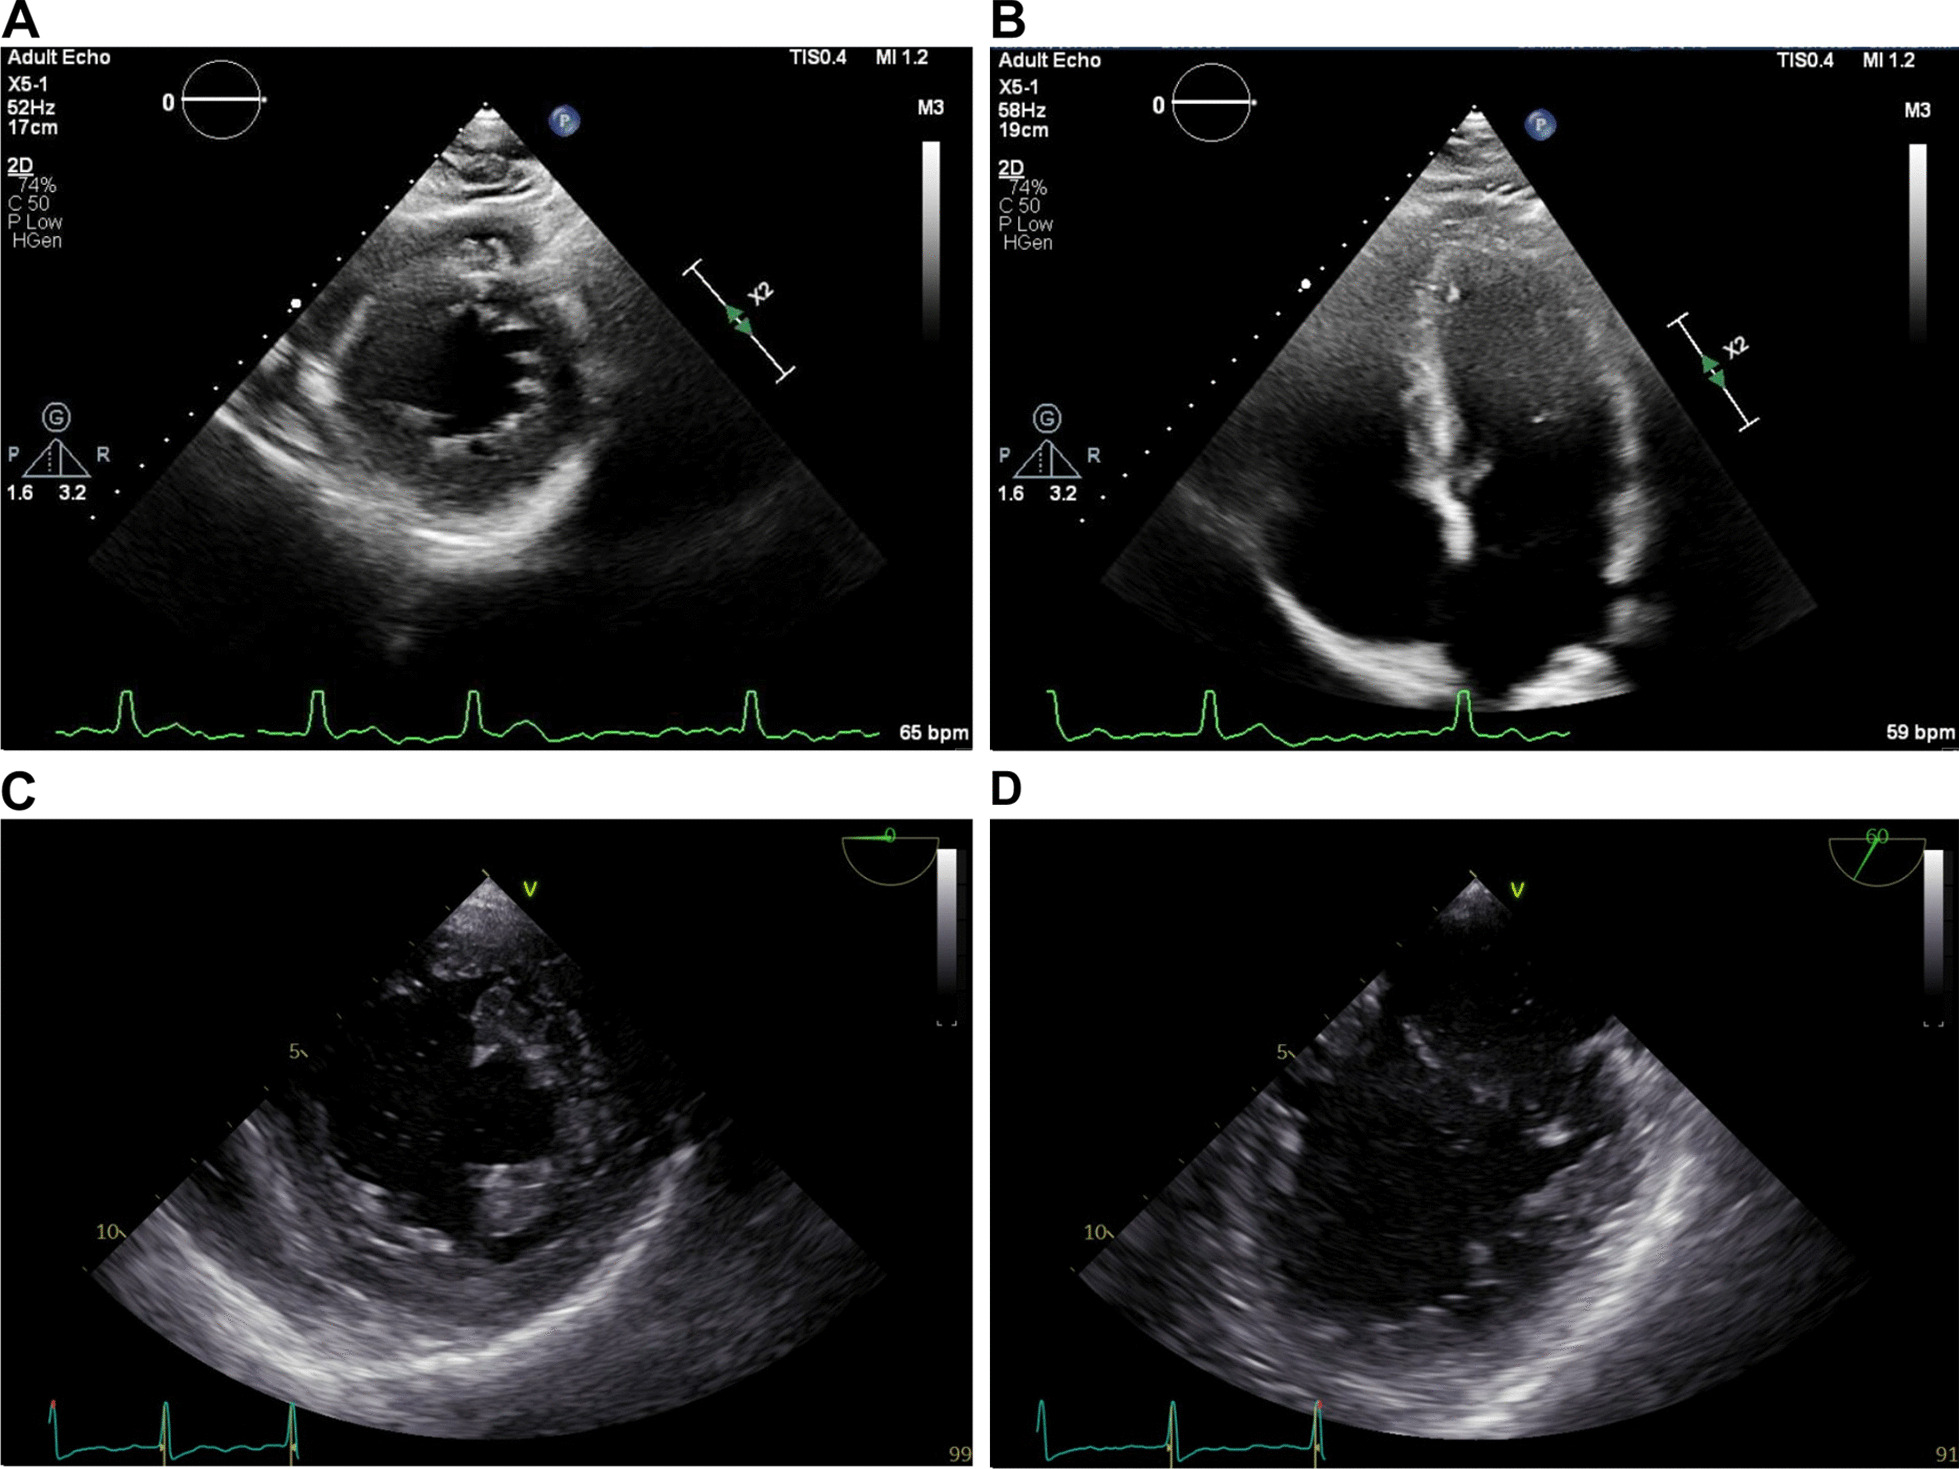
Transthoracic (A, B) and transesophageal (C, D) echocardiogram pictures. A Parasternal short-axis view; (B) apical four chamber view; C transgastric midpapillary short-axis view; D midesophageal commissural view
